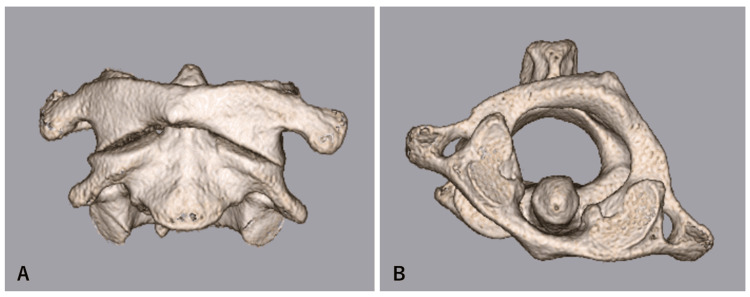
Three-dimensional computed tomography (3D-CT) of the atlas and axis The atlas and axis are viewed from the front (A) and from above (B). In addition to the plain CT (Figure 1), the atlas is rotated to the right relative to the axis and fixed. No expansion of the atlantodental interval (ADI) is observed.
